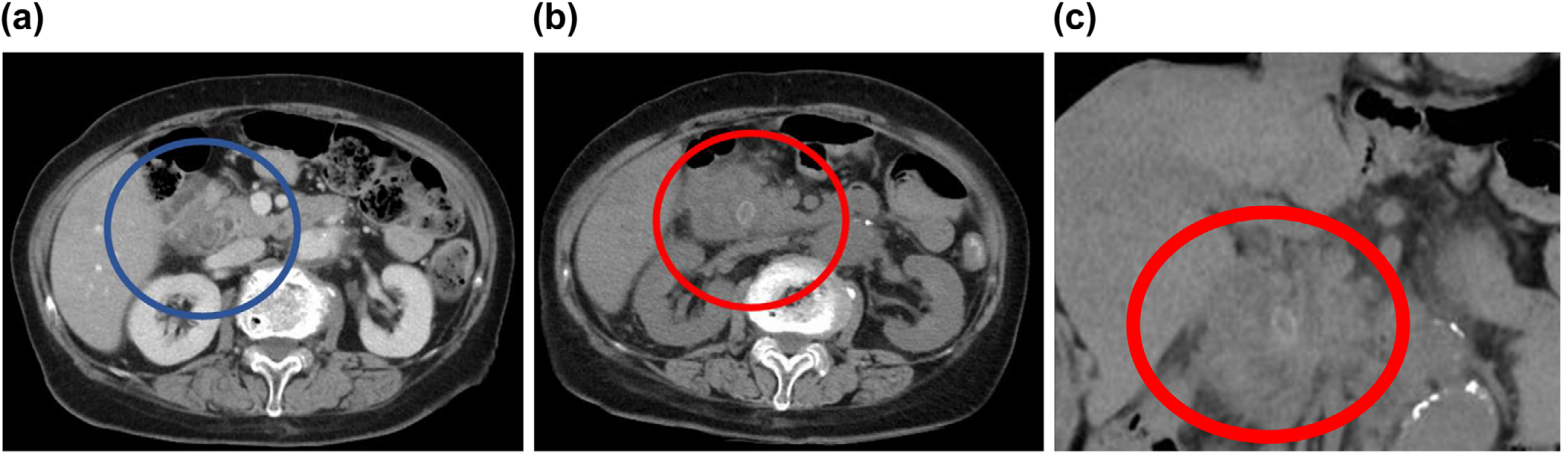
(a) Contrast‐enhanced computed tomography (CT) scan on admission revealed edematous wall thickening of the duodenum. (b) A re‐examination of plain CT images revealed the presence of calcified duodenal diverticular calculus, leading to a diagnosis of duodenal diverticulitis with parapapillary calculus. (c) The plain CT coronal section showed the calcified duodenal diverticular calculus as a diverticulum located beside the duodenal papillae, slightly toward the mouth on the side of the pancreatic head.
